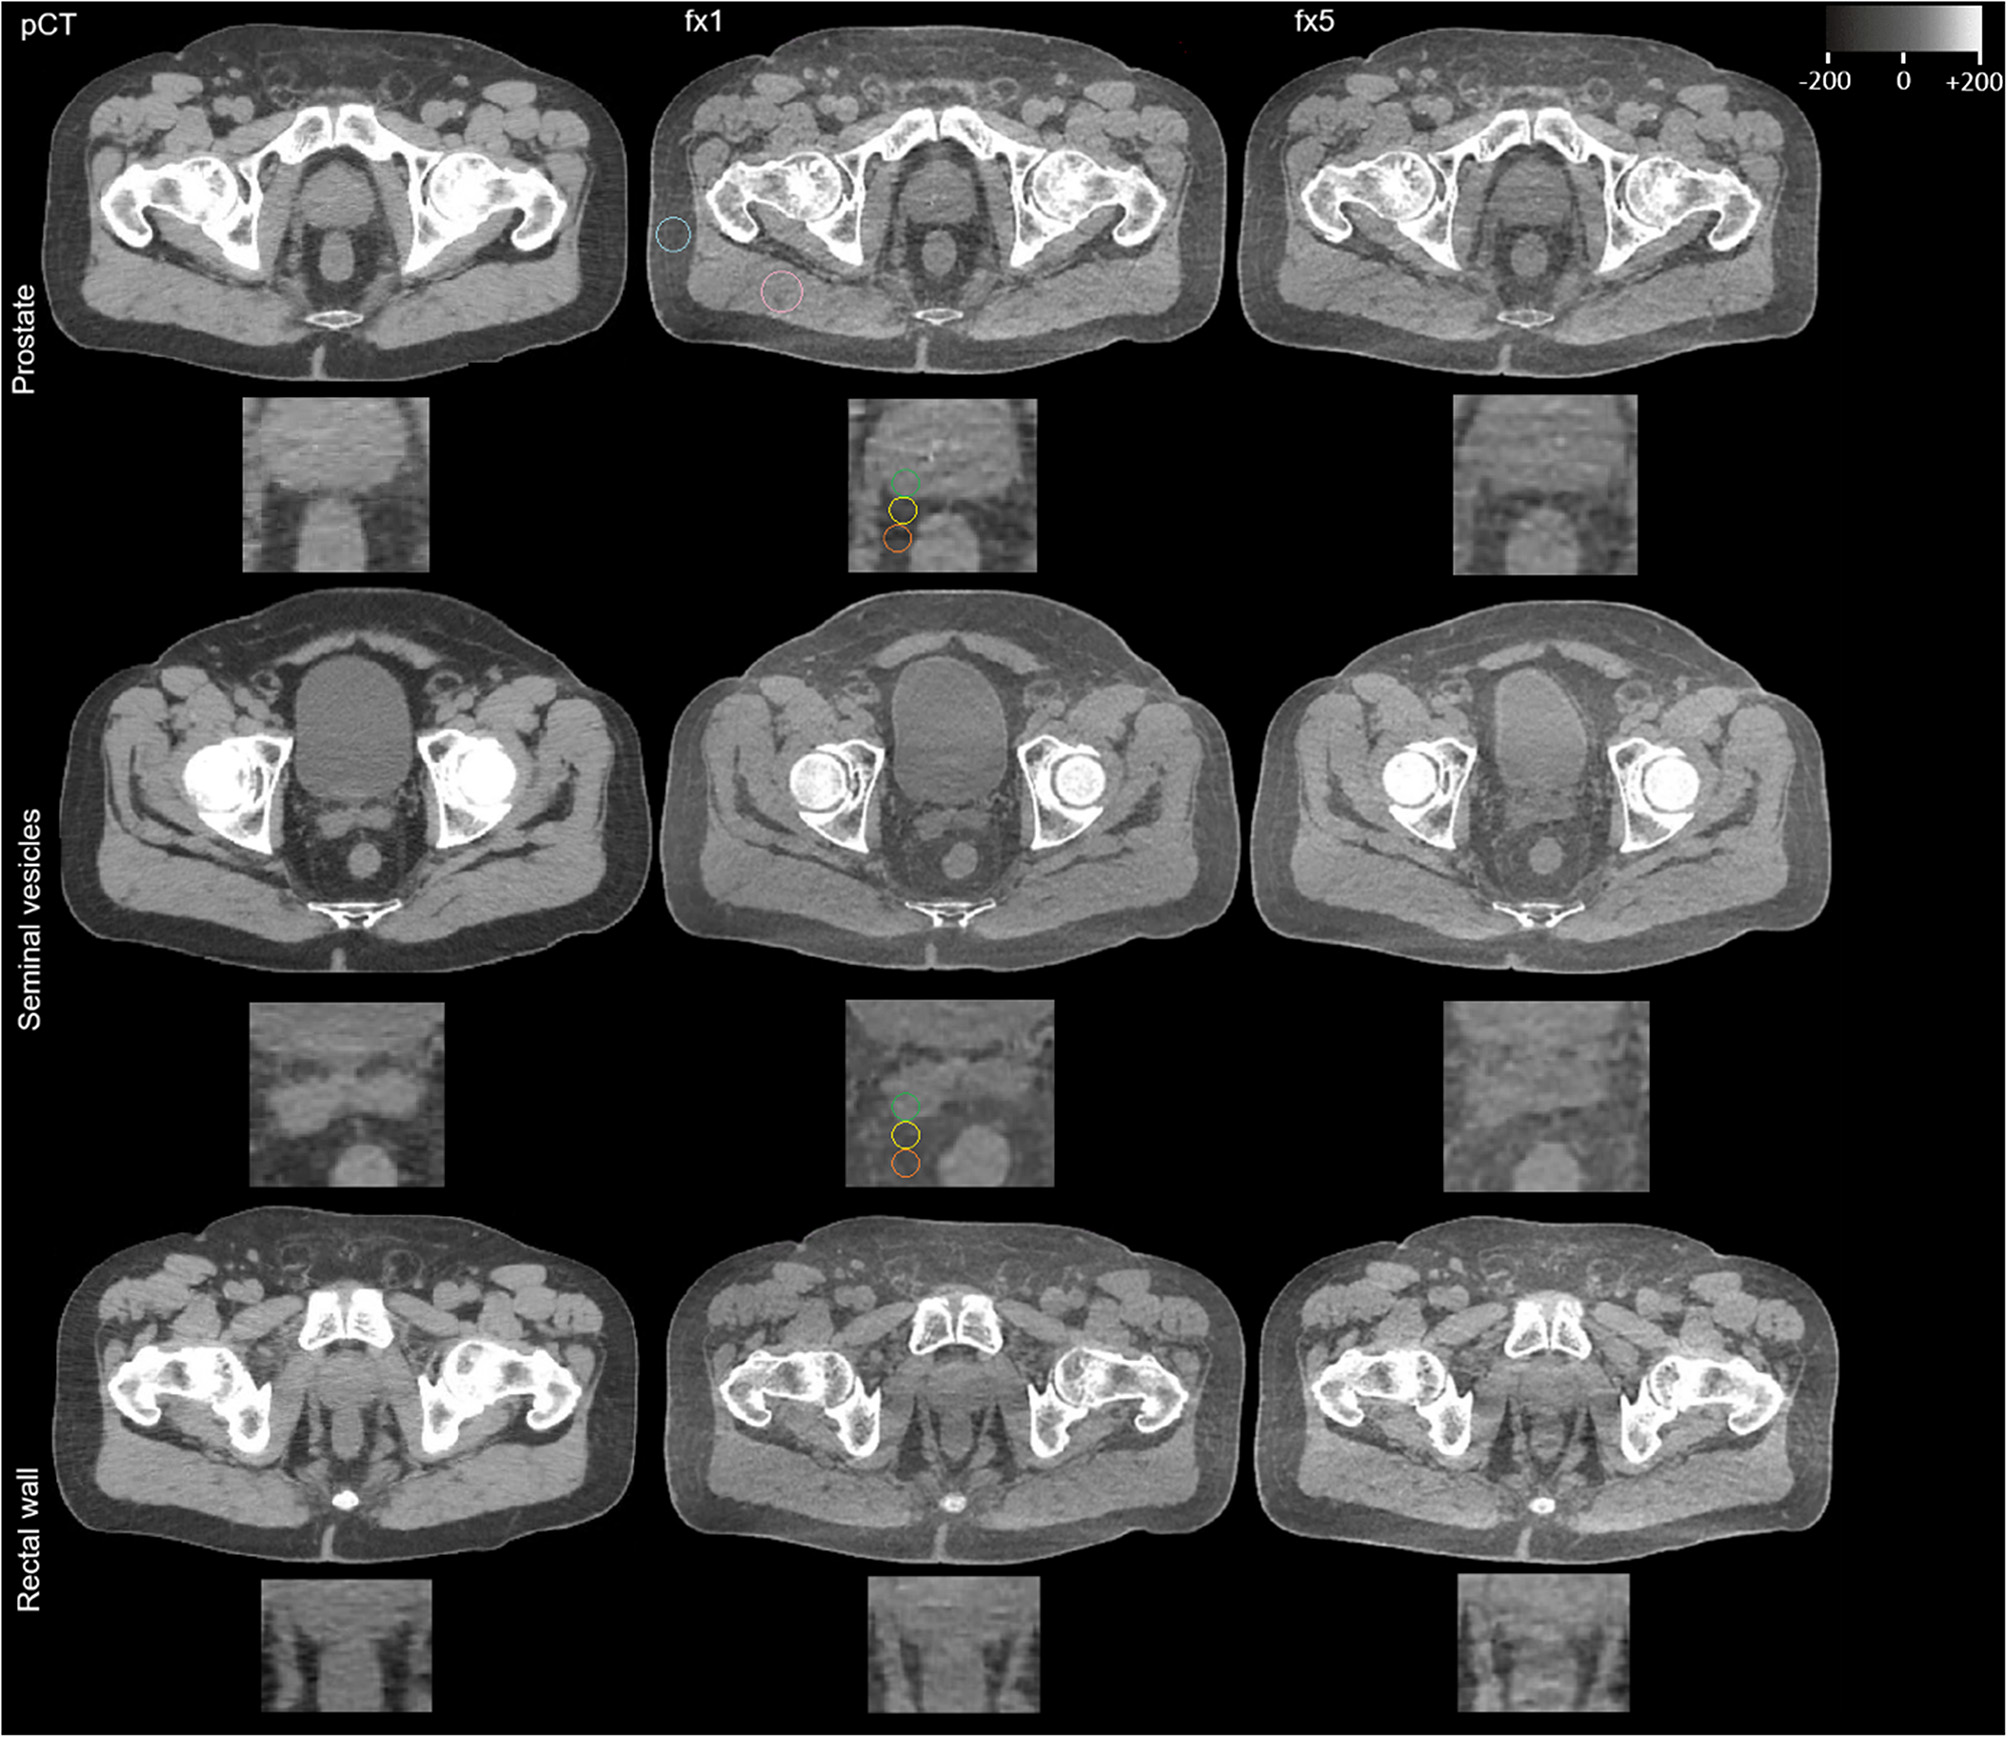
Comparison of Pelvic Imaging. Shown is the comparative analysis of pelvic imaging quality across treatment fractions. The planning computed tomography (pCT) scan (Brilliance BigBore, Philips) serves as the baseline for evaluating HyperSight™ CBCT scans on an Ethos^®^ linear accelerator (Varian, Siemens Healthineers) from the first (fx1) and fifth (fx5) fractions, with particular focus on the prostate-seminal vesicle complex and anterior rectal wall. Following key findings emerge: First, while pCT and fx1 CBCT show comparable contour sharpness, there is a noticeable decline in contour sharpness with fx5, particularly at the prostate-rectal interface. This trend is quantified by strategically placed regions of interest (ROIs) at tissue interfaces at the height of both target regions prostate and seminal vesicles (ROI1 = green, outer organ tissue/ROI2 = yellow, fat at the organ border/ROI3 = orange, peripheral fat) (see Results Sect. “Quantitative patient based analysis: longitudinal change of CT Attenuation in fractional hCBCT at the target interface”). Structural reference ROIs (turquoise/pink) in pelvic fat and gluteus maximus muscle are used to calculate the quantitative parameters of image quality (see Results Sect. “Quantitative patient based analysis: image quality parameters”)
